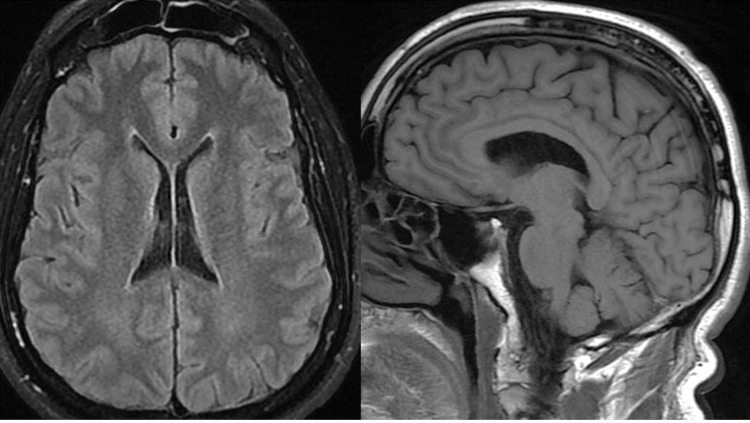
Brain MRI resonance with contrast shows no cerebral abnormality.
